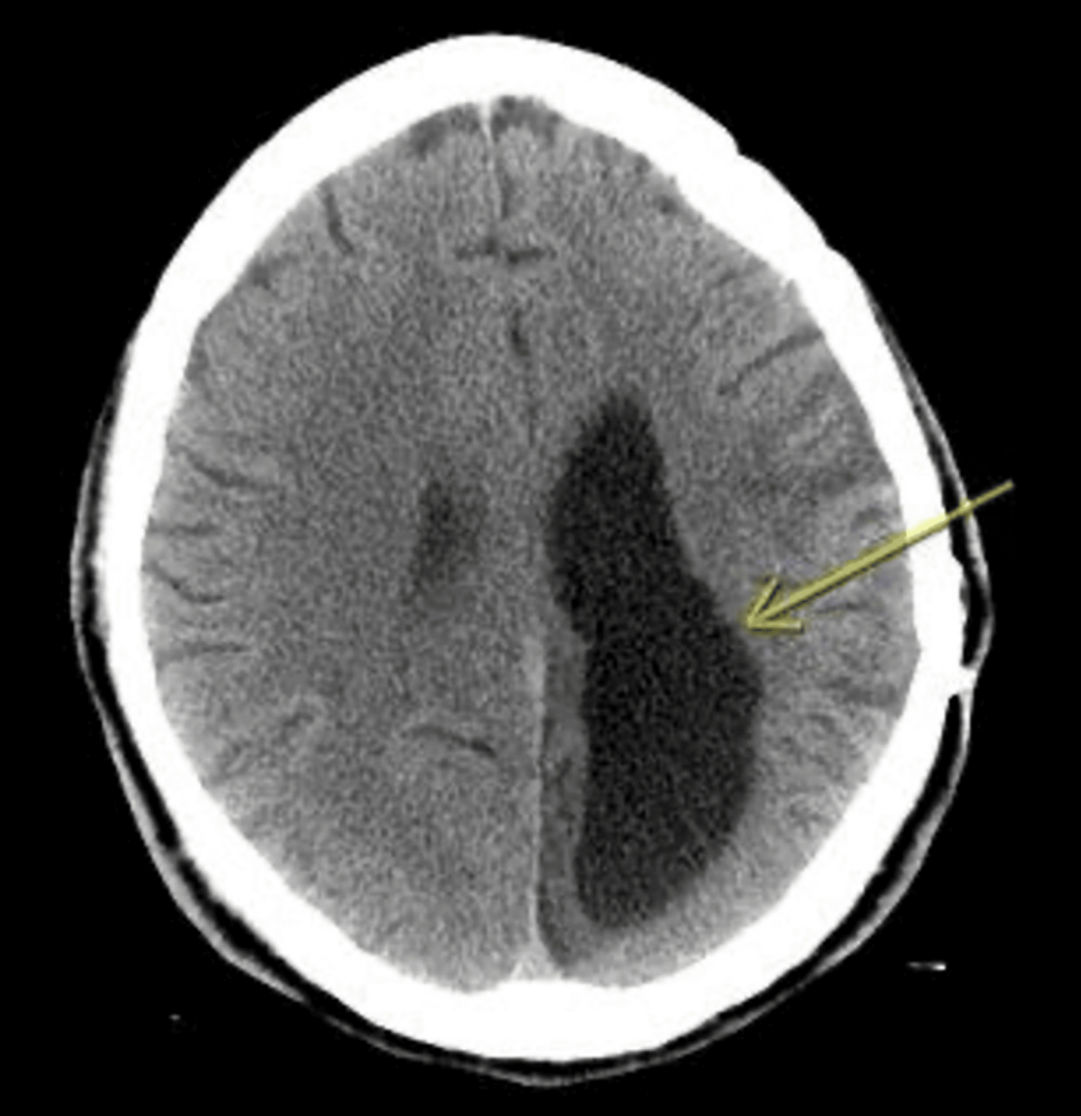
CT brain without contrast showed left frontal and temporal encephalomalacia and left occipital parietal chronic infraction.
